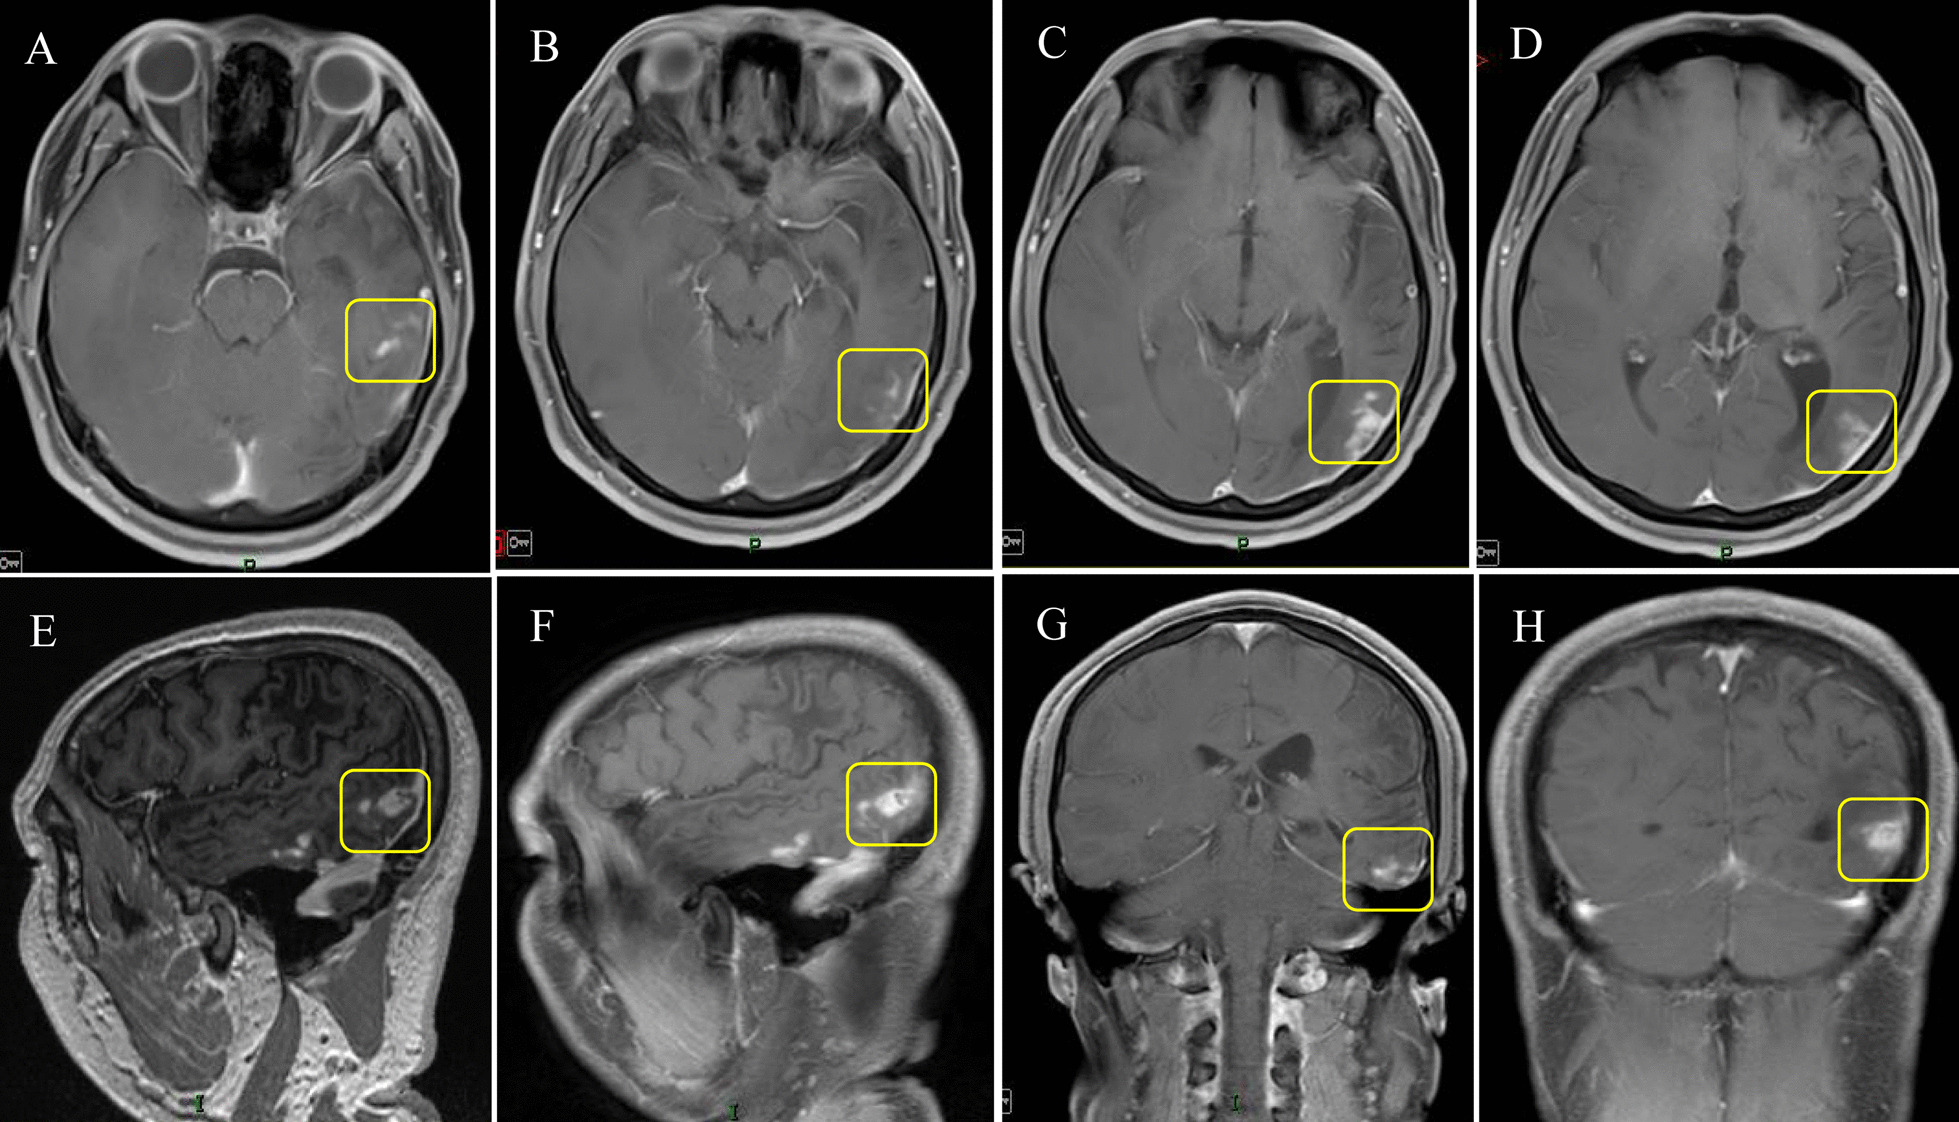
Scar tissue hyperplasia on contrast-enhanced MRI. A–H Contrast-enhanced MRI shows hyperplasia of scar tissue produced by the long-term parasitism of Sparganus, as shown in the yellow box
